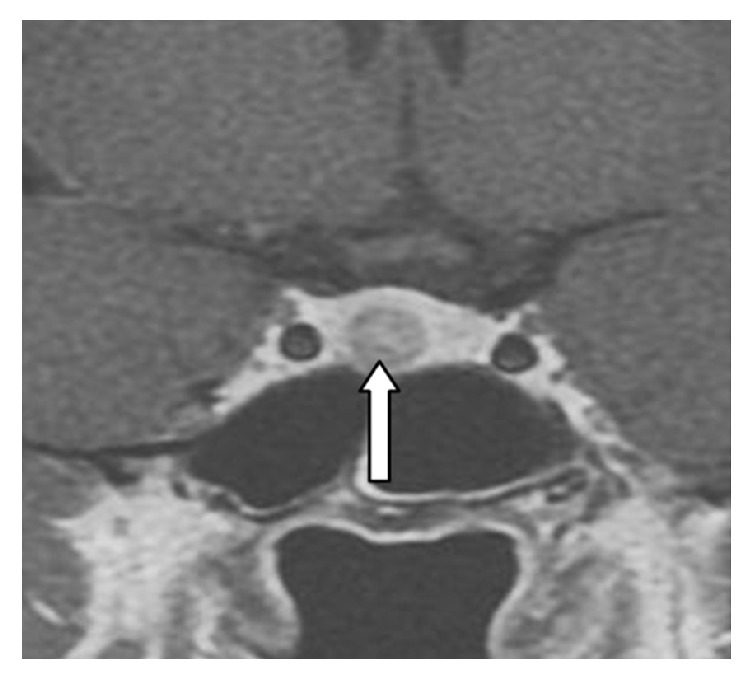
MRI of the pituitary microadenoma.
